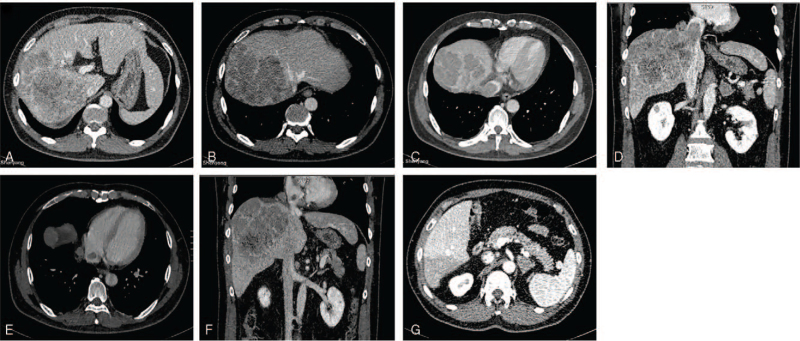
A, B: Enhanced CT revealed a mass tumor in the right lobe of liver, also invaded part of the middle hepatic vein; C, D: The tumor thrombus in the inferior vena cava; E, F: The tumor thrombus in the right atrial; G: Left adrenal gland metastasis. CT = computed tomography.
